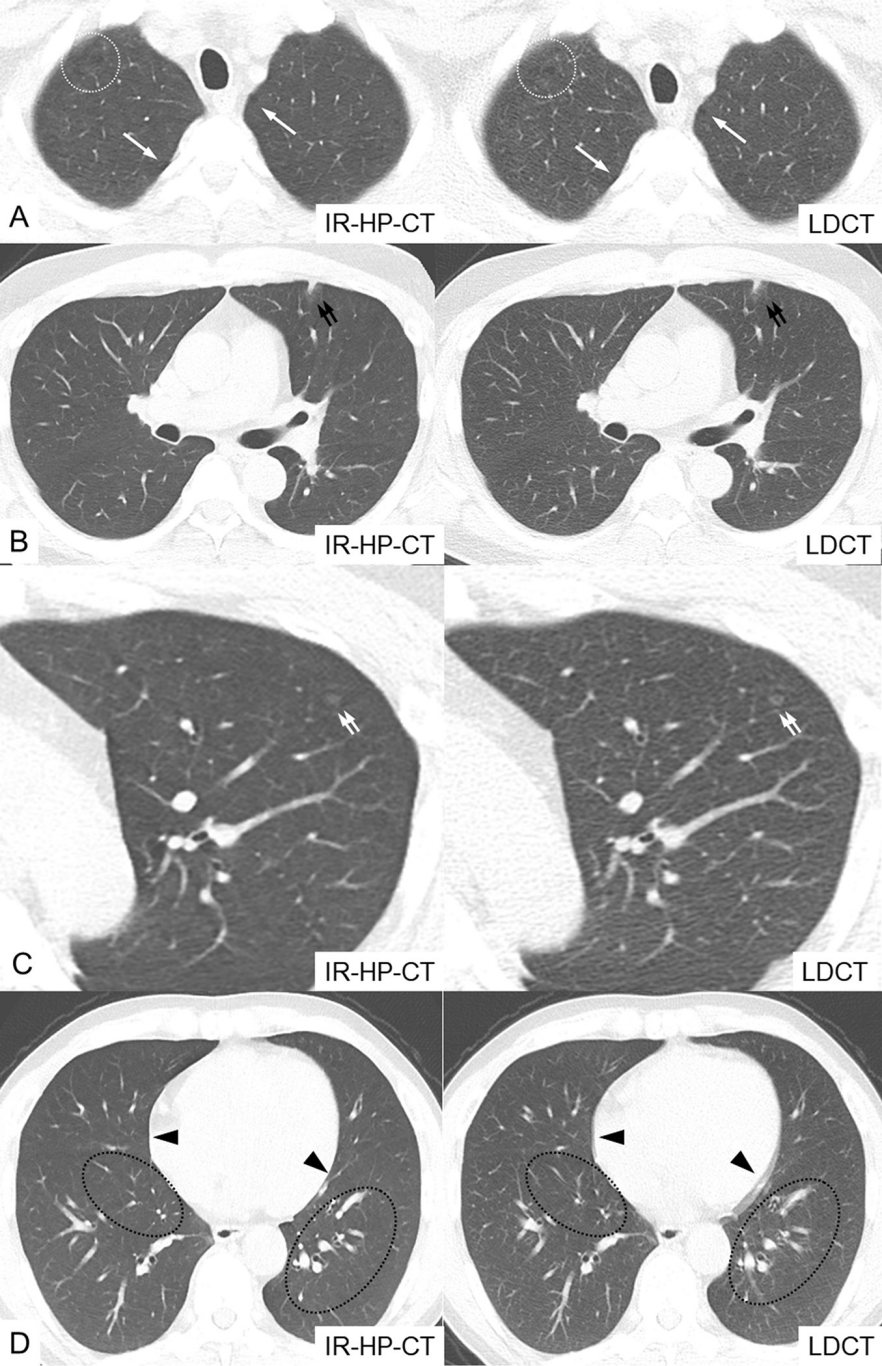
Various pulmonary lesions and cardiac pulsation artifacts detected on IR-HP-CT and LDCT. (A) Small bullae in both upper lobes (white arrows) and centrilobular emphysema in the right upper lobe (dotted circle). (B) Focal nodular consolidation with peripheral ground glass opacity in the subpleural area of the left upper lobe (paired black arrows). (C) A 3-mm-diameter ground glass opacity in the left upper lobe (paired white arrows). (D) IR-HP-CT revealed no cardiac pulsation (4 points), but LDCT was associated with blurring of both cardiac margins (2 points) at the time of cardiac pulsation (black arrowheads). Compared with IR-HP-CT, the LDCT scans exhibited bronchial wall and pulmonary vessel blurring artifacts attributed to cardiac pulsation in the pericardiac areas of both lung fields (dotted ovals).
